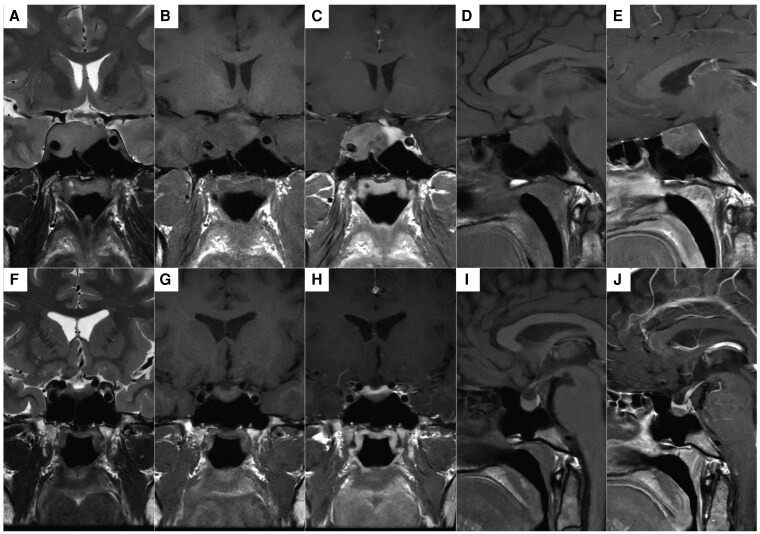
Standard (Tier 1a) MRI in 2 patients with somatotroph adenomas. 1.5T pituitary MRI in 2 patients with somatotroph adenomas. (A-E) Middle-aged man with a macroadenoma demonstrating Knosp 4 extension to the right cavernous sinus: (A) coronal T2W FSE, (B) coronal T1W SE, (C) coronal T1W SE post-gadolinium, (D) sagittal T1W SE, and (E) sagittal T1W SE post-gadolinium. The adenoma is hyperintense to the gray matter of the temporal lobe on T2W sequences (panel A) and shows poor enhancement when compared with the normal gland (left sella), which is best appreciated on the T1W SE post-gadolinium coronal image (panel C). (F-J) Young woman with an inferiorly located mesoadenoma without parasellar extension: (F) coronal T2W FSE, (G) coronal T1W SE, (H) coronal T1W SE post-gadolinium, (I) sagittal T1W SE, and (J) sagittal T1W SE post-gadolinium. The adenoma is hypointense to the gray matter of the temporal lobe on T2W sequences (panel F) and shows poor enhancement when compared with the normal gland (superiorly), which is best appreciated on the T1W SE post-gadolinium images (panels H and J). Abbreviations: FSE, fast SE; MRI, magnetic resonance imaging; SE, spin echo; T, Tesla; T1W, T1-weighted; T2W, T2-weighted.
